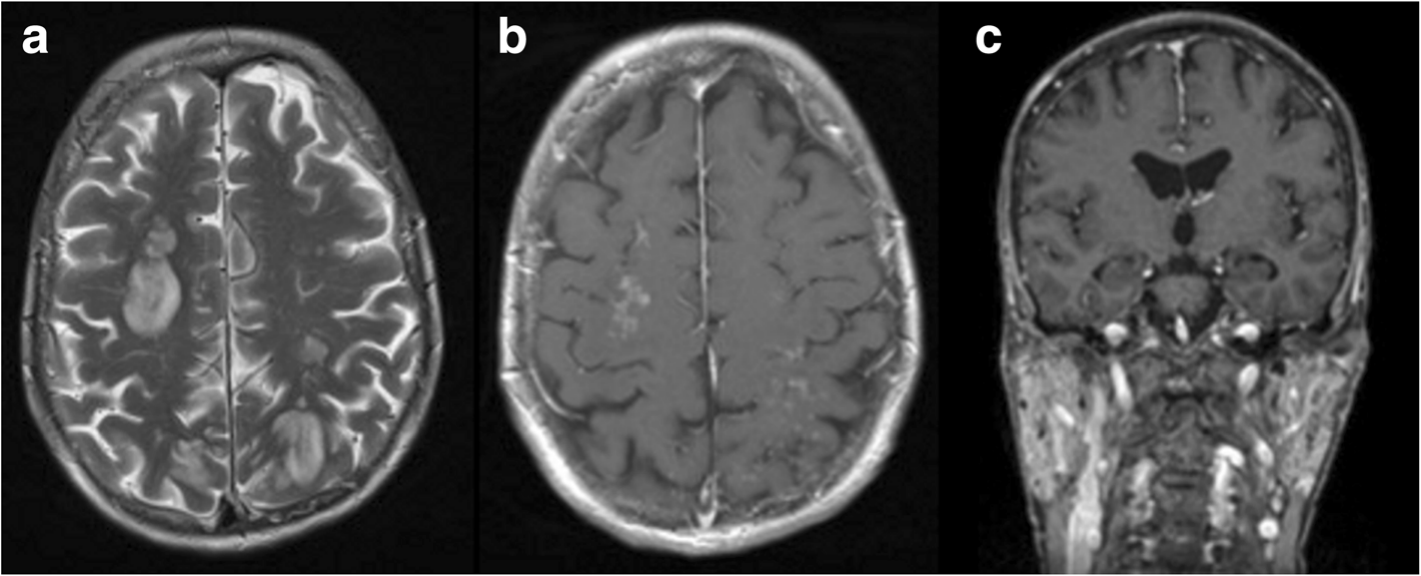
a–c Initial magnetic resonance imaging (MRI) of the brain. The initial MRI scan showed multiple disseminated, bihemispheric, supratentorial cortical, and subcortical hyperintense white matter lesions in the axial T2-weighted (3 mm) images, especially in the frontal, parieto-occipital, and occipitotemporal lobes (a). The white matter hyperintensities revealed contrast enhancement with a diffuse, fine granular pattern (b; axial T1-weighted image after contrast application, 3 mm). The parotid glands were diffusely enlarged on both sides with multiple small cystic areas and tiny contrast-enhancing nodules (c; coronal T1-weighted MPR after contrast application, 1 mm)
